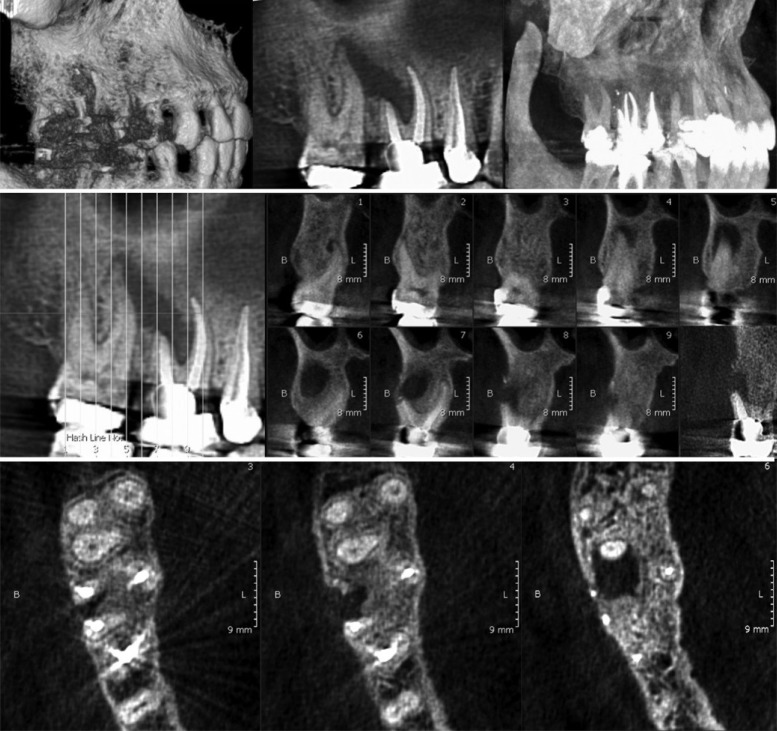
CBCT views showing obvious relationship between the mesiobuccal root of the maxillary second molar and the lesion
